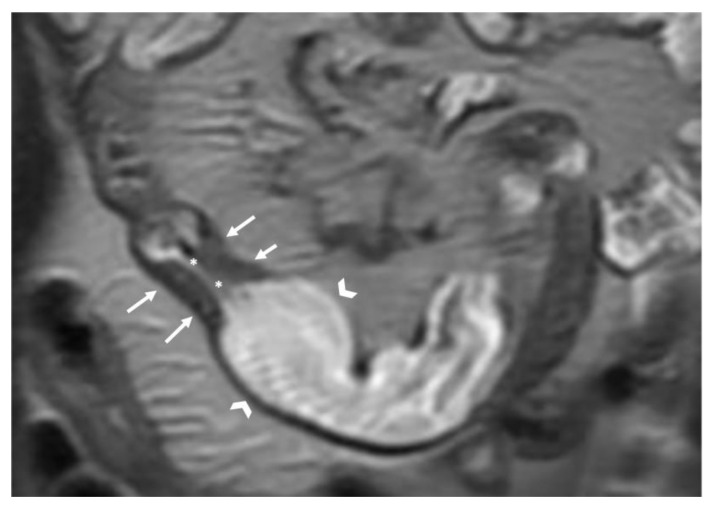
Coronal T2-weighted image shows bowel wall thickening (arrows), determining lumen stenosis (asterisks) and marked upstream dilatation (arrowheads).
